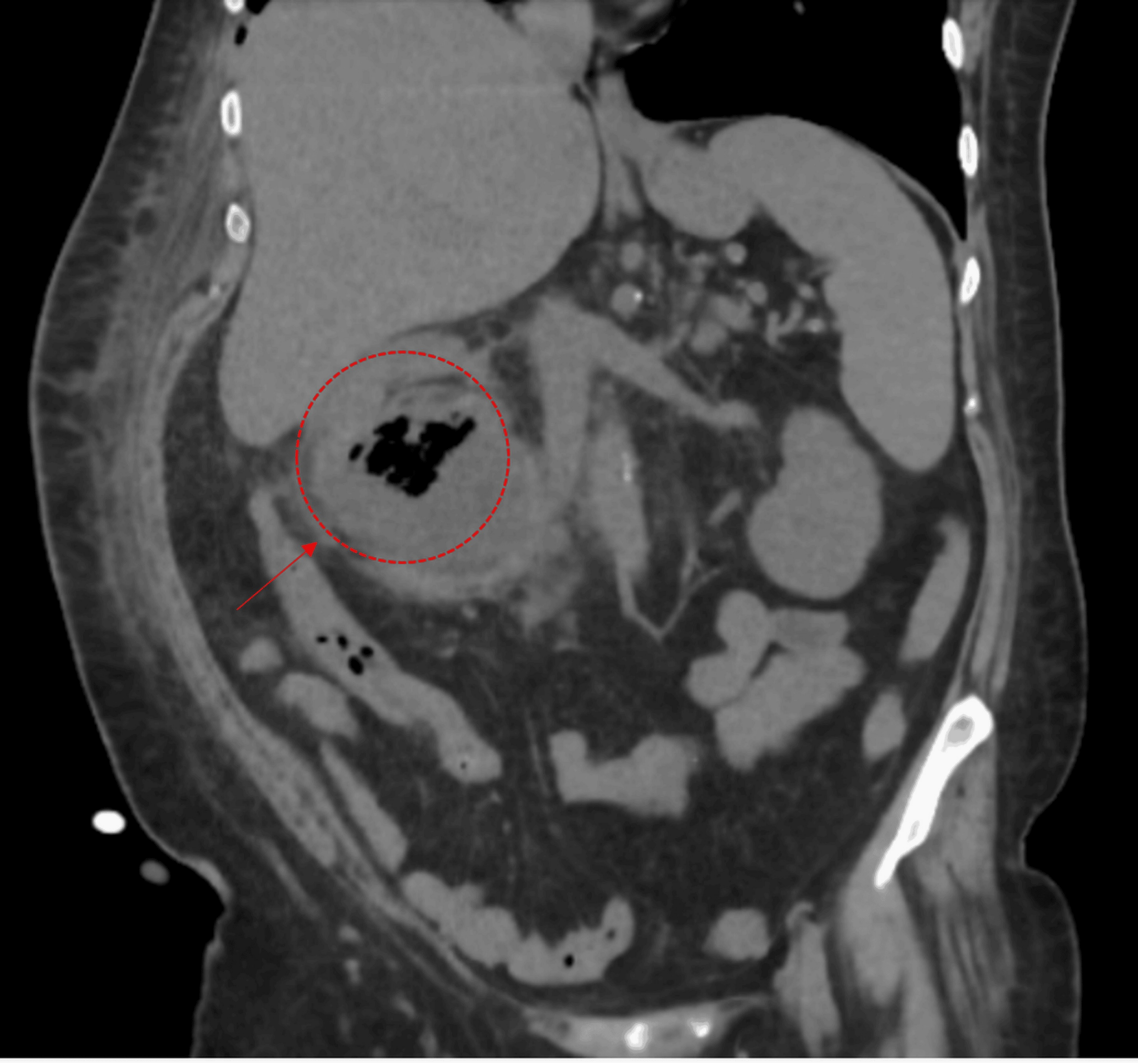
Contrast-enhanced CT image showing a large retroperitoneal abscess in the right renal fossa, extending into the psoas muscle, marked with a red arrow
